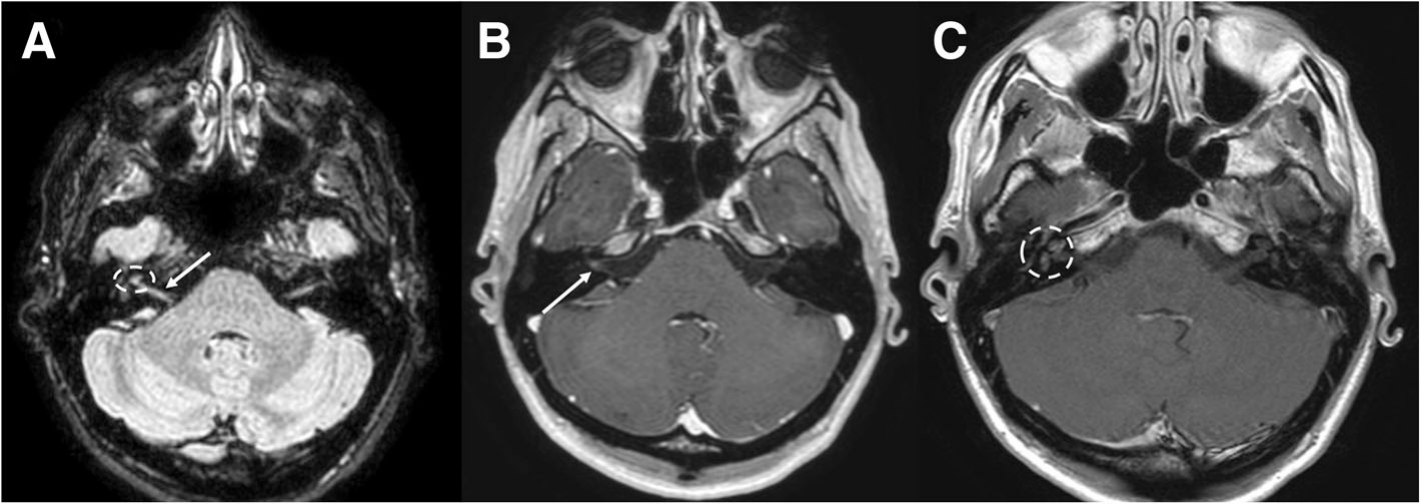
Vestibular neuritis. MRI FLAIR (a) and T1-weighted post-gadolinium (b, c) axial images show mild hyperintensity (a, arrow) and enhancement (b, arrow) of the right vestibular nerve and right labyrinth (a, c, dotted circles)
